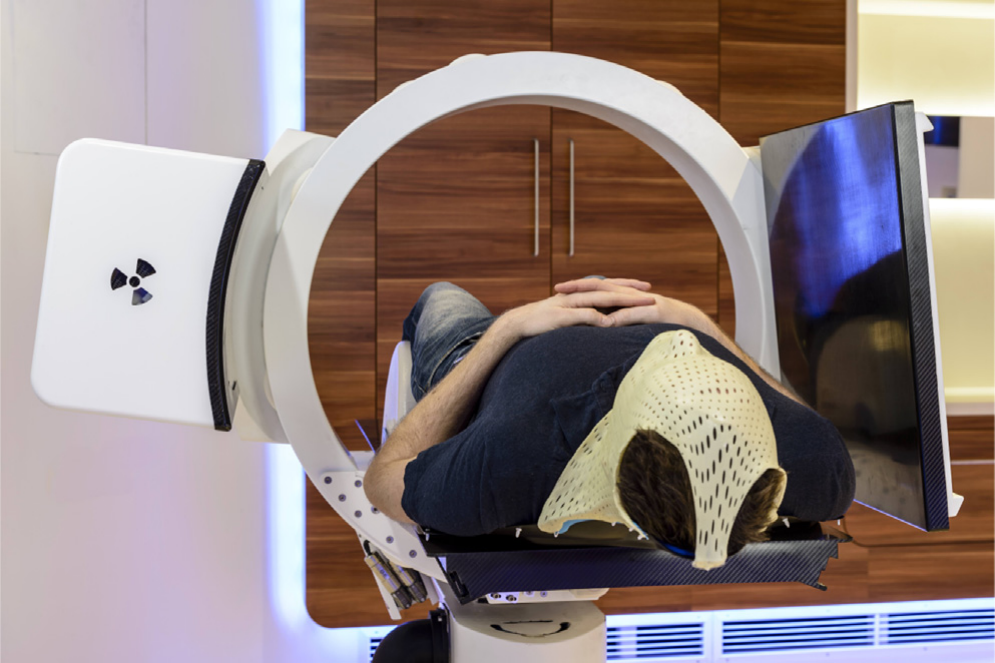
ImagingRing system as installed in a clinical treatment room at MedAustron ion therapy facility, depicting X-ray source and detector mounted on the ring. [(c) Kästenbauer/Ett].
